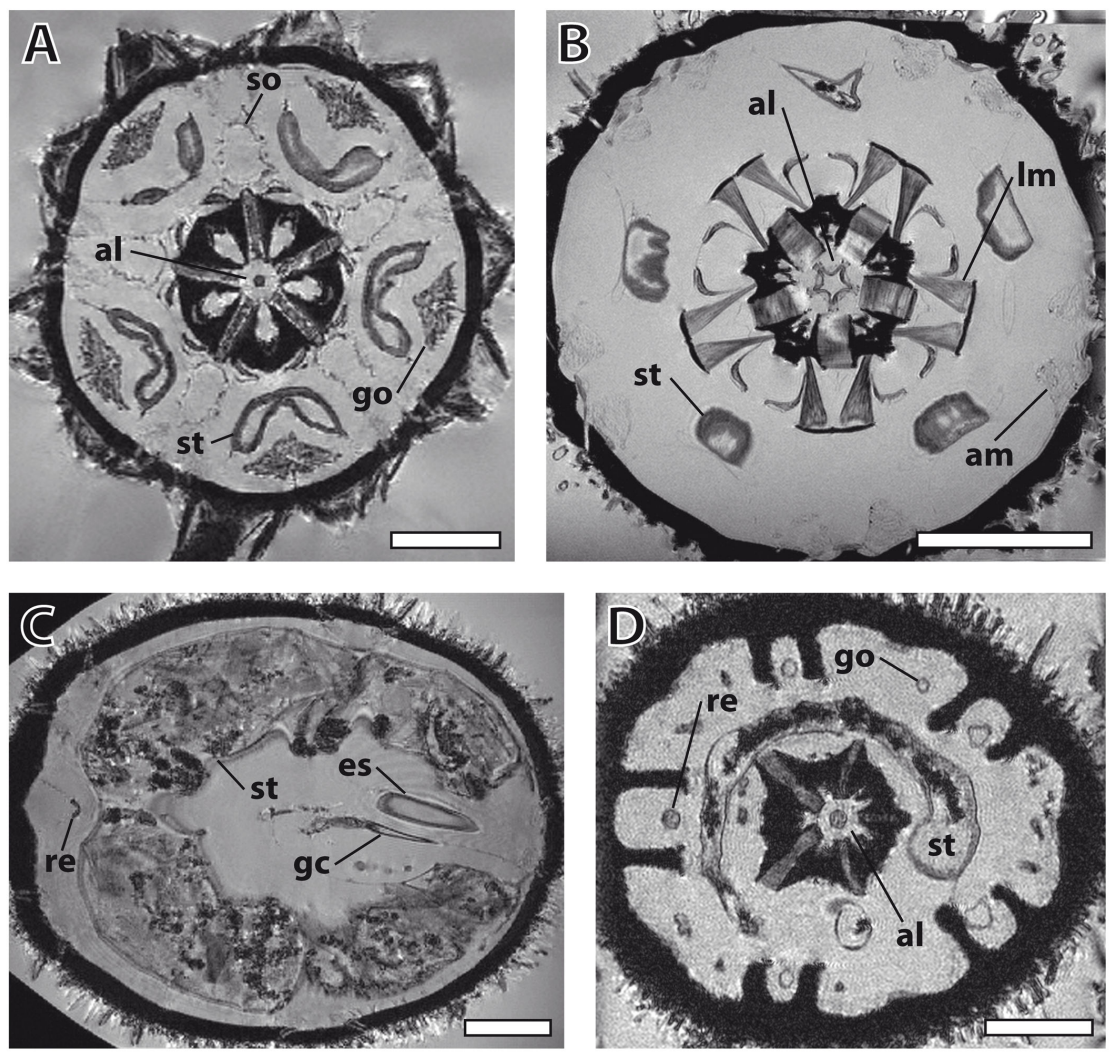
Selected horizontal magnetic resonance imaging sections of different sea urchin species taken from Additional files 3, 4, 5, 6. (A) *Eucidaris metularia*. Resolution: (81 μm)^3^. Aristotle's lantern, gonads, Stewart's organs, and stomach can be seen. (B) *Psammechinus miliaris*. Resolution: (44 μm)^3^. Aristotle's lantern, lantern muscles, stomach, and ampullae are visible. (C) *Echinoneus cyclostomus*. Resolution: (86 μm)^3^. Digestive tract with oesophagus, gastric caecum, stomach, and rectum are shown. (D) *Echinocyamus pusillus*. Resolution: 20 × 18 × 18 μm^3^. Aristotle's lantern, gonads, stomach, and rectum are represented. Scale bar: (A)-(C) 0.5 cm; (D): 1 mm. al, Aristotle's lantern; am, ampulla; es, oesophagus; gc, gastric caecum; lm, lantern muscle; re, rectum; so, Stewarts' organ; st, stomach.
